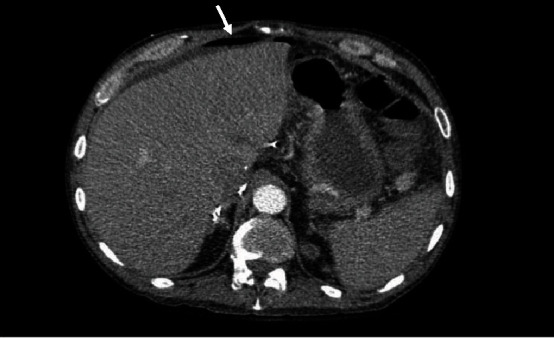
Axial abdominal CT angiogram showing free air in peritoneal cavity (white arrow) secondary to perforated duodenum.
